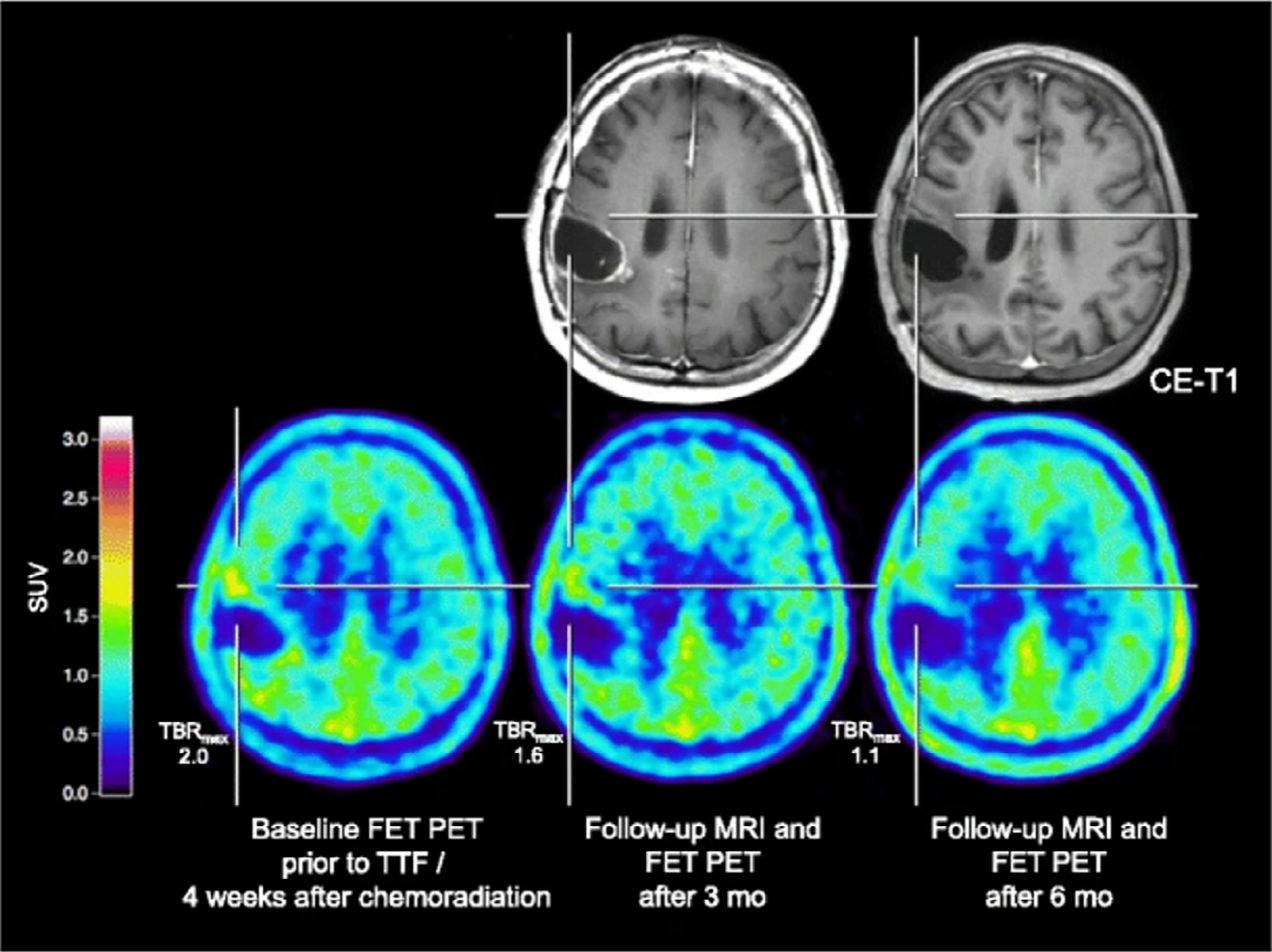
Images before TTFields and during follow‐up of a 73‐year‐old patient with GBM. Due to immune thrombocytopenia, only TTFields were applied 4 weeks after completion of radiotherapy with concomitant TMZ. Before TTFields, baseline FET PET images showed a slight increase in metabolic activity (TBRmax 2.0, TBRmean 1.6) without spatial counterpart contrast enhancement. Sequential FET PET images at 3 and 6 months follow‐up showed a decrease in metabolic activity, as evidenced by a decreased tumor‐to‐brain ratio. Compared to MRI T1 images, FET PET images showed clearer lesions. Adapted permission from (Ref. 100).
